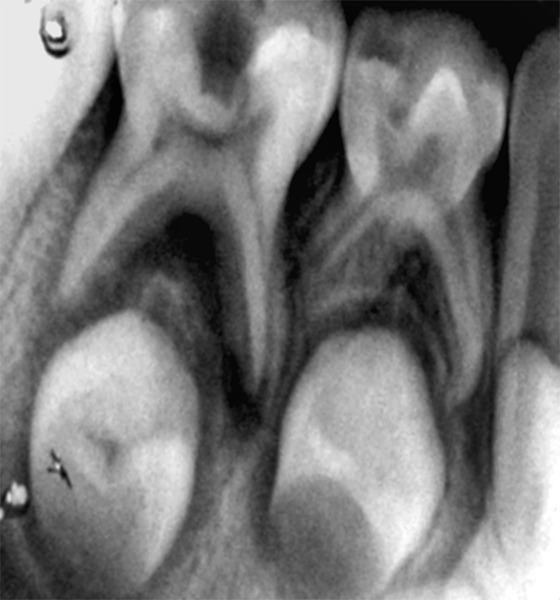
Preoperative radiograph in relation to 74
